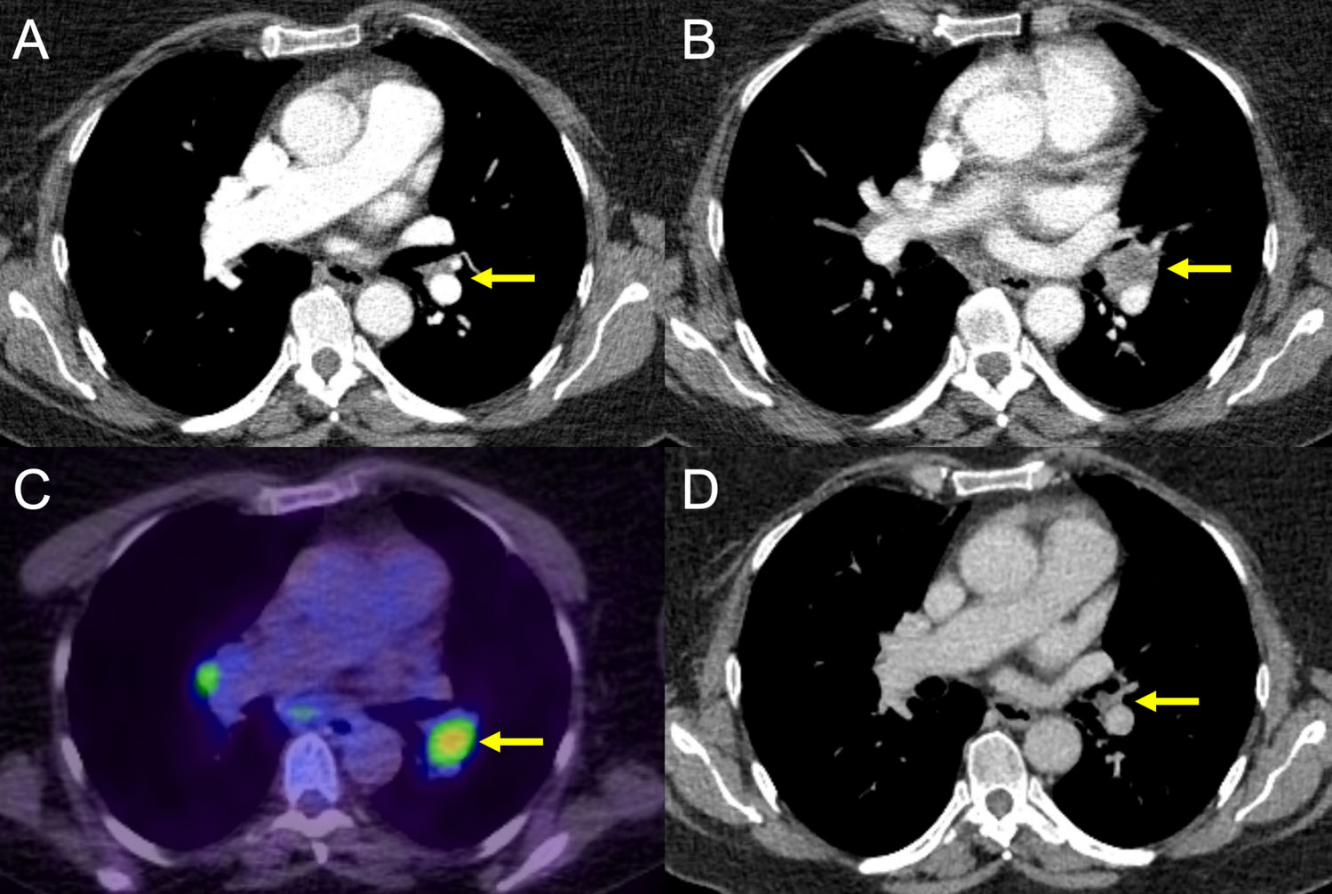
67-year-old female patient (No. 5) experiencing hepatic and mediastinal lymph node disease progression under anti-PD1 therapy. A shows an axial contrast-enhanced CT (Angio-CT) of the thorax from March 2023 with no evidence of definitive mediastinal or parahilar lymph node metastases. B shows an axial contrast-enhanced CT of the thorax from the follow-up in August 2023 with clear evidence of mediastinal and parahilar lymph node metastases. C shows the corresponding PET-CT. D shows the follow-up CT in May 2025 after combined ICI and TACE therapy showing a complete response of the extrahepatic metastases, indicating an abscopal effect (the yellow arrows indicate the target lesion in each image)
